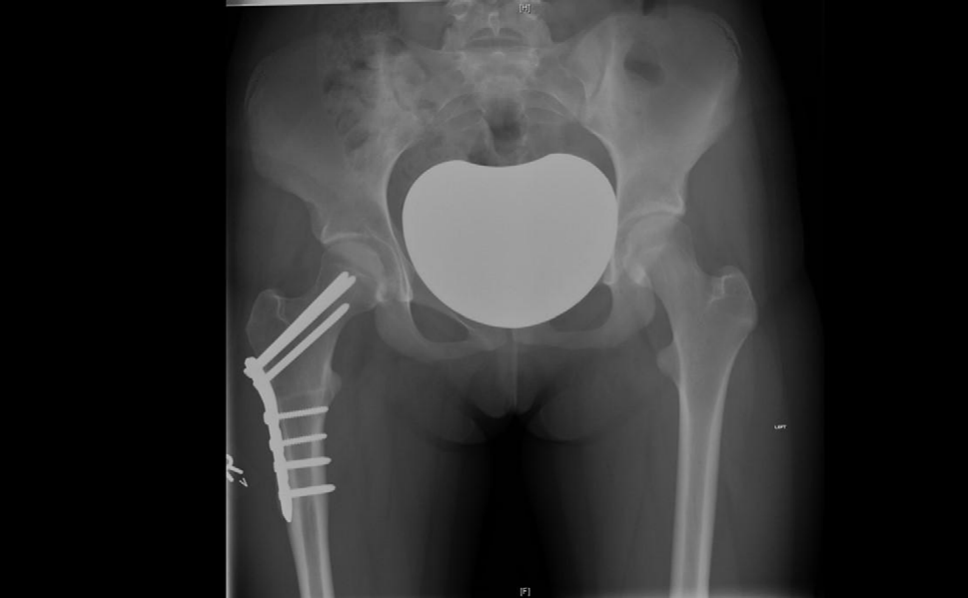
Radiograph of pelvis and hips, at three months postderotation proximal femoral osteotomy on right side.
